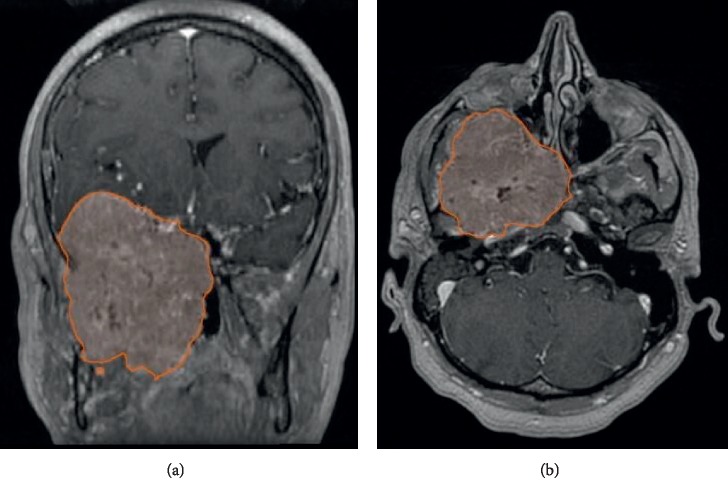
T1 Enhanced-MRI: tumor lesion located at the right skull base with 163.2 cm^3^ volume.
